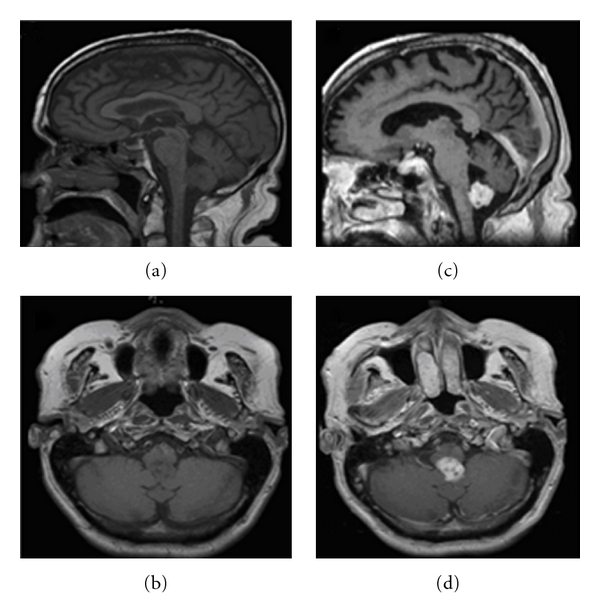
Sagittal and axial pre- and Postcontrast MR images. (a) Sagittal image without gadolinium showing a homogenous lesion in the fourth ventricle. (b) Axial image without gadolinium showing the lesion. (c) Sagittal postcontrast image showing a heterogeneous enhancement of the lesion. (d) Axial postcontrast also showing heterogeneous enhancement.
